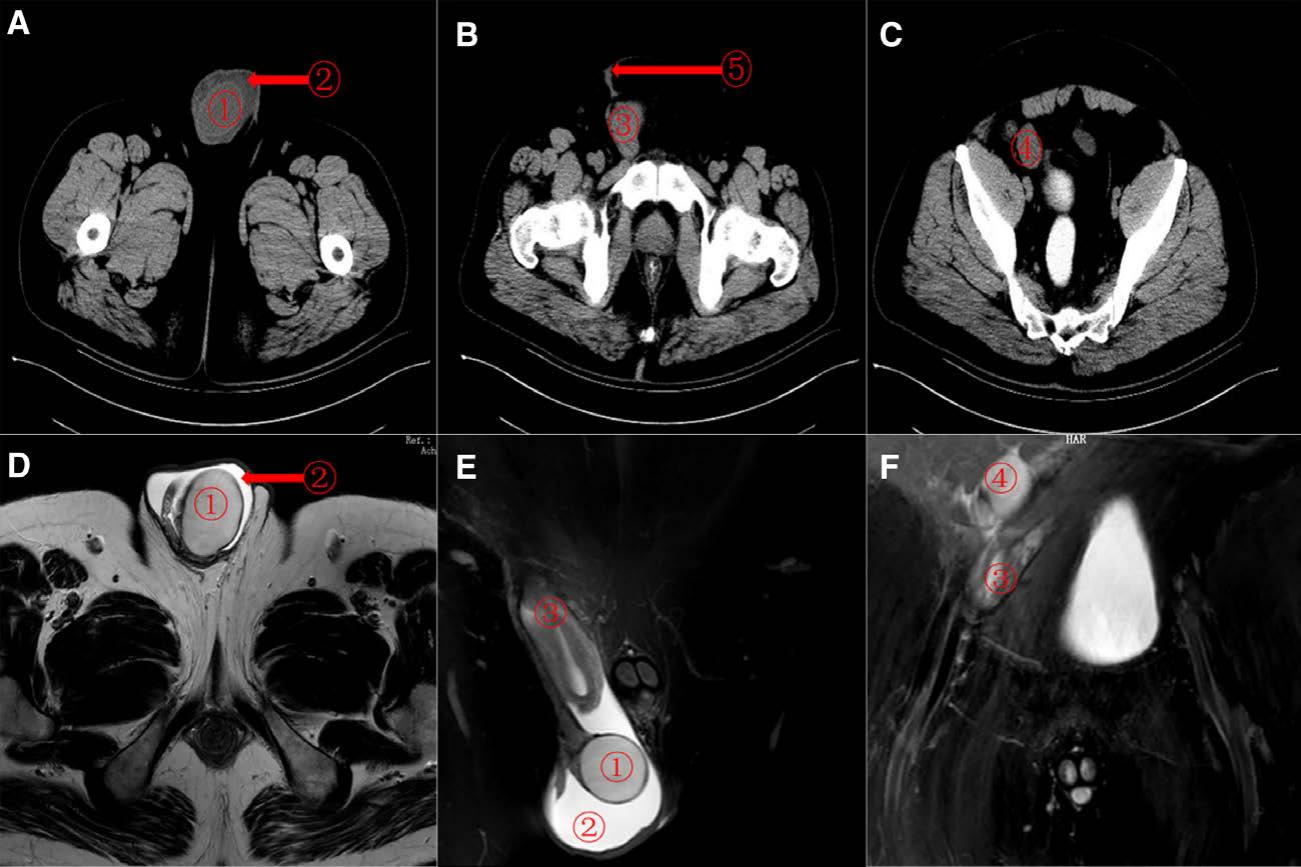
Preoperative CT (A–C) and MRI (D–F) images. ① Right testicle in normal position. ② Right testicular hydrocele. ③ Right inguinal canal tissue, mullerianduct tissue and left seminal vesicle gland? ④ Left ectopic testis in the right groin region. ⑤ Scar tissue in the right groin area. CT = computerized tomography, MRI = magnetic resonance imaging.
